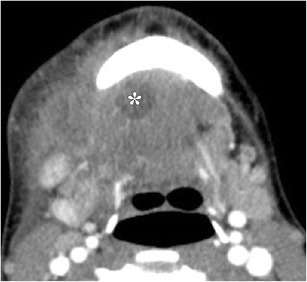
Sublingual/submandibular space abscess. Axial contrast-enhanced CT image through the floor of the mouth demonstrates extensive subcutaneous fat stranding and inflammatory changes centred within the right submandibular and sublingual spaces. A well-circumscribed fluid-attenuating collection is present within the right sublingual space consistent with early abscess formation (*)
